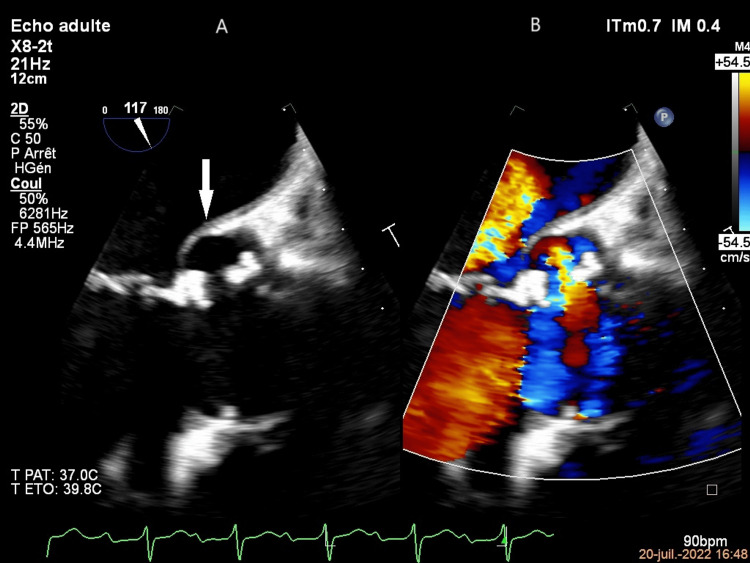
TEE mid-esophageal long-axis view (A) TEE without Doppler color showing the abscess (white arrow). (B) TEE with Doppler color showing the fistulae between the abscess and the aortic root TEE: transesophageal echocardiogram
